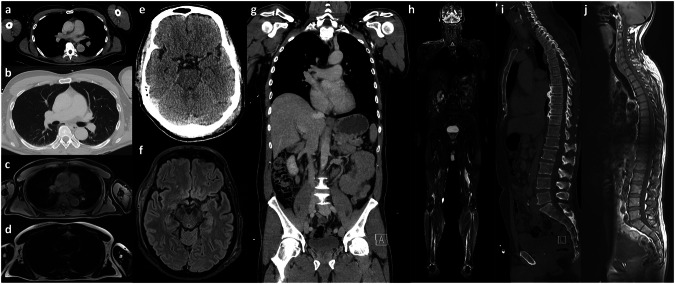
Example of whole-body imaging in CT and MRI. A 59-year-old patient who had a bike accident not wearing a helmet. Transverse CT slices of the thorax in soft tissue window (a) and lung window (b) and corresponding MRI in DIXON sequence with (c) and without fat saturation (d). Transverse CT-slices of the brain in soft tissue window (e) and corresponding FLAIR-sequence (f), both depicting a temporal galea hematoma on the right side and a temporooccipital galea hematoma on the left side. Coronal reconstruction of the venous phase of thorax and abdomen in soft tissue window (g) and composed coronal TIRM-sequences of the whole patient (h). Sagittal CT reconstruction of the spine in the bone window (i) and composed sagittal T1-weighted MRI images (j)
